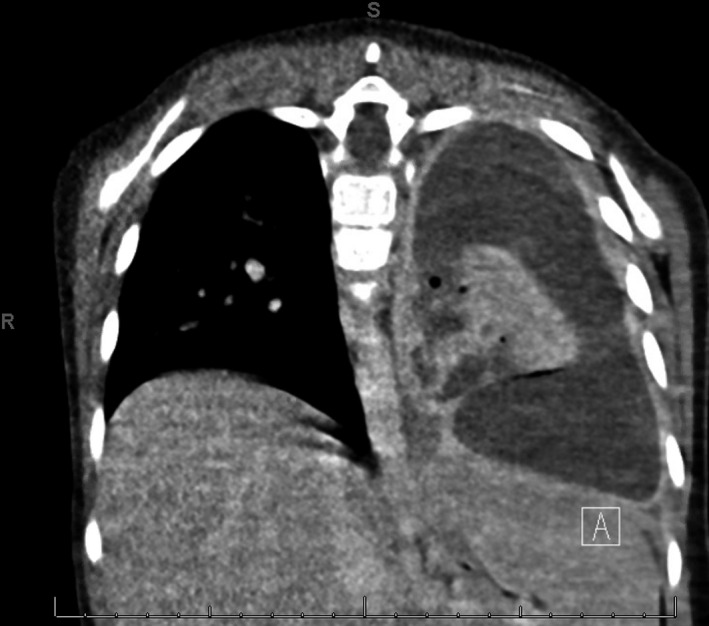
Chest CT coronal view demonstrating left lower lung necrosis with atelectasis and extensive effusion
